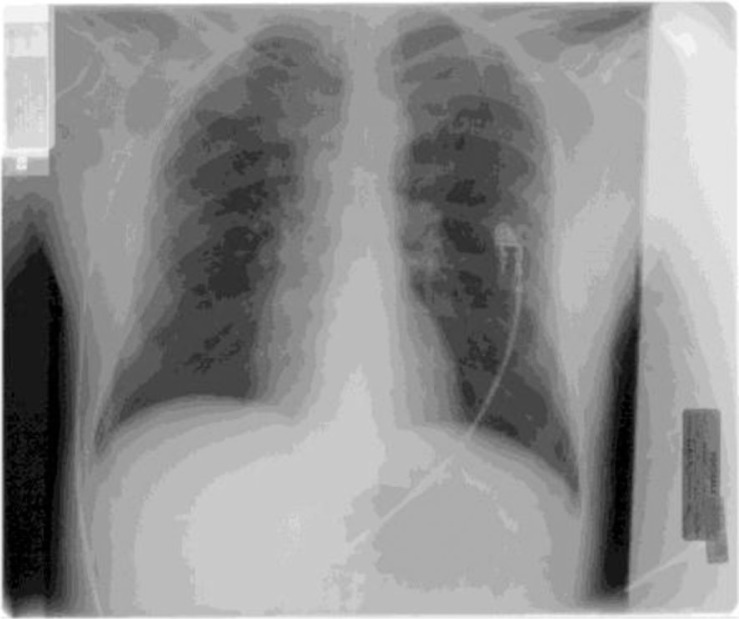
Chest radiograph showing no underlying infiltrates or cardiomegaly.
